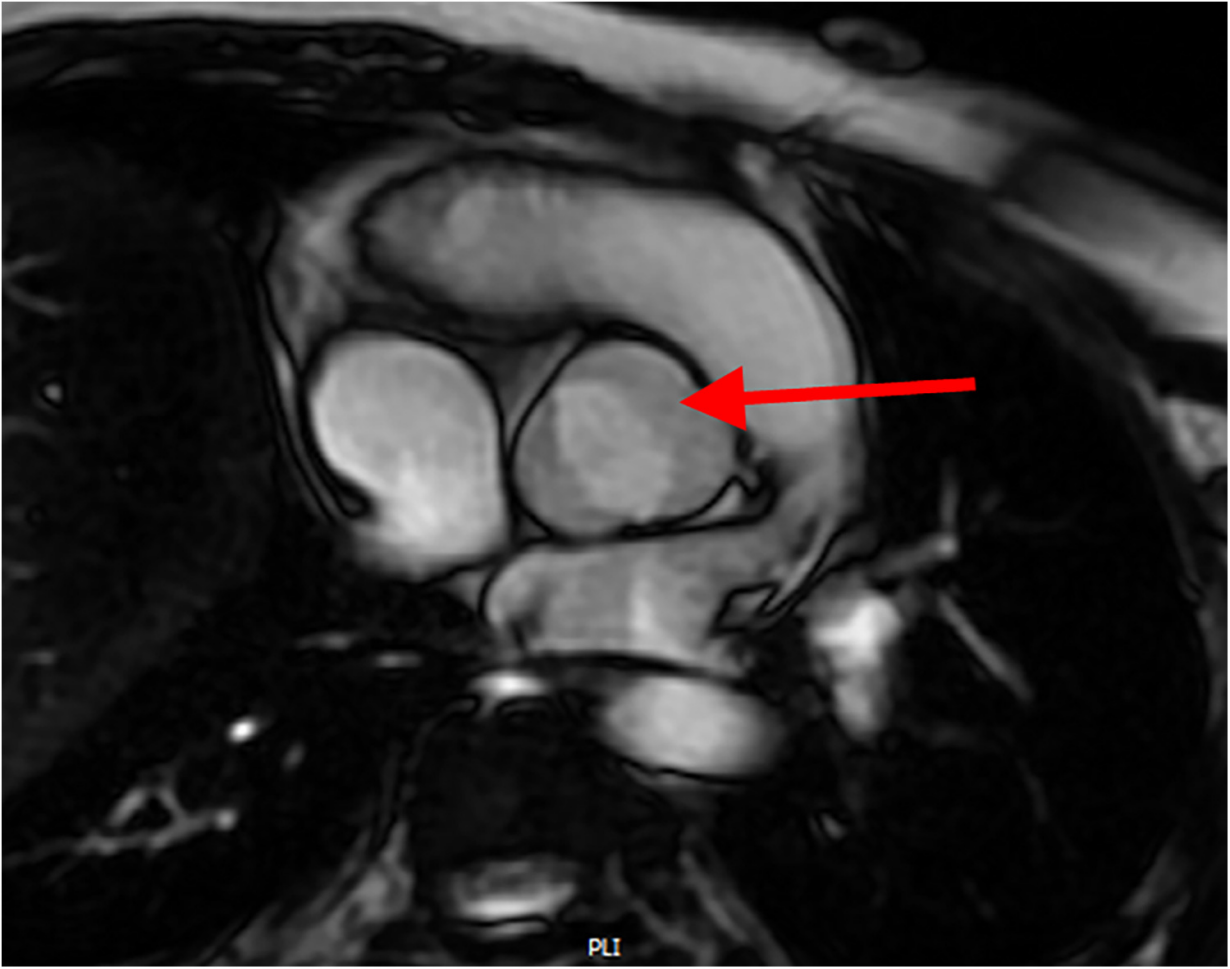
Cardiac MRI showing a bicuspid aortic valve with fusion of the right and left coronary cusps (arrow).
